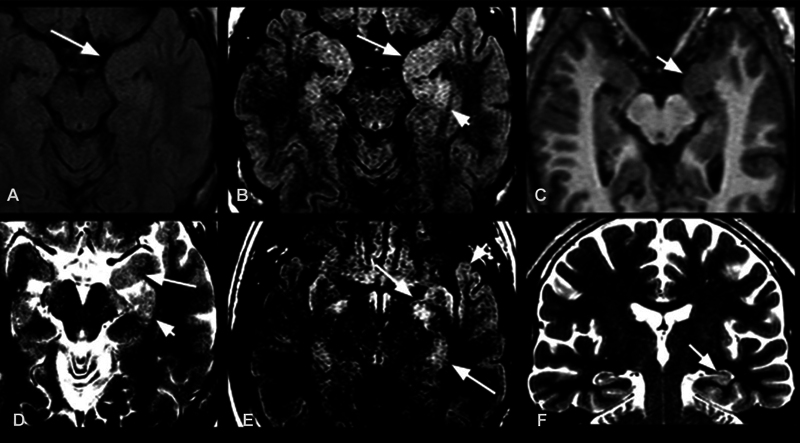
Brain MRI showing mild enlargement of the left amygdala with subtle FLAIR and T2 hyperintensity (A: standard window). Findings are more evident when window settings are adjusted (arrows in B and D). T1 sequence shows minimal hypointensity (C). The left hippocampus demonstrates FLAIR and T2 hyperintensity (short arrow in D; long arrows in E and F). (Author's property).
